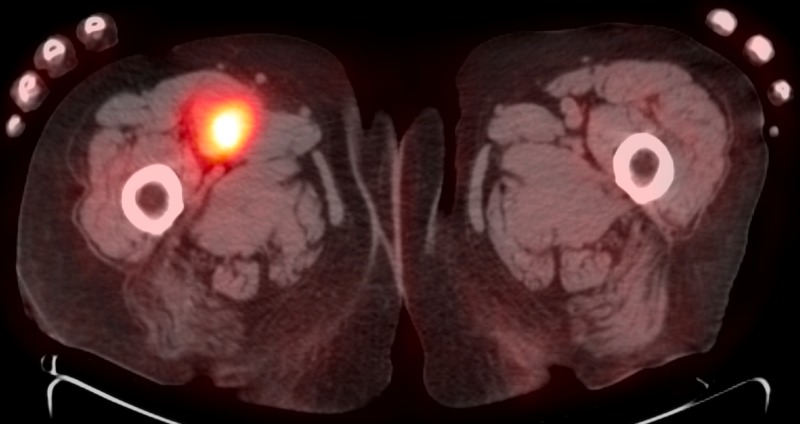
PET/CT of August 25, 2015 positron emission tomography-computed tomography: PET/CT
